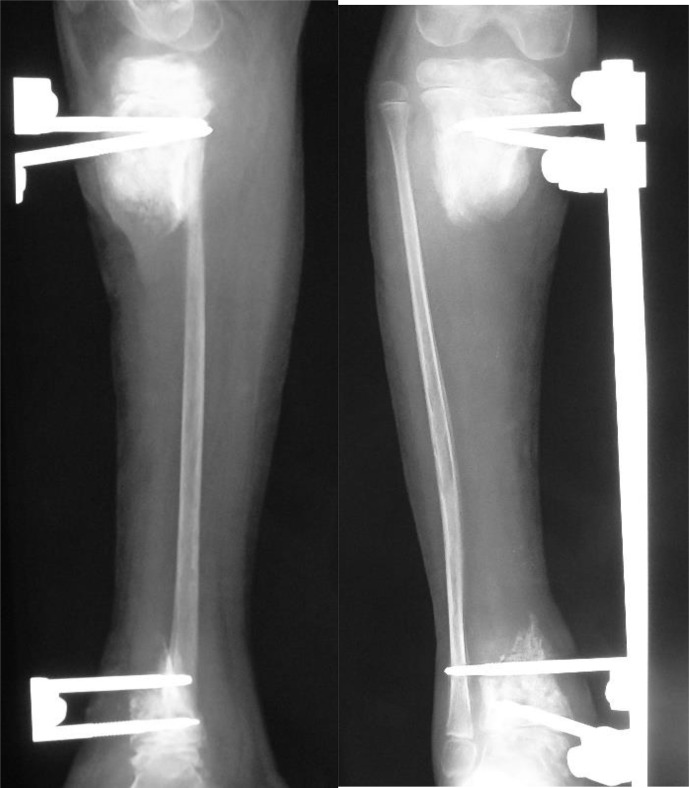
Radiographs showing the post-sequestrectomy bone defect in a 7-year-old child, who presented with exposed sequestrum, loss of soft tissue
